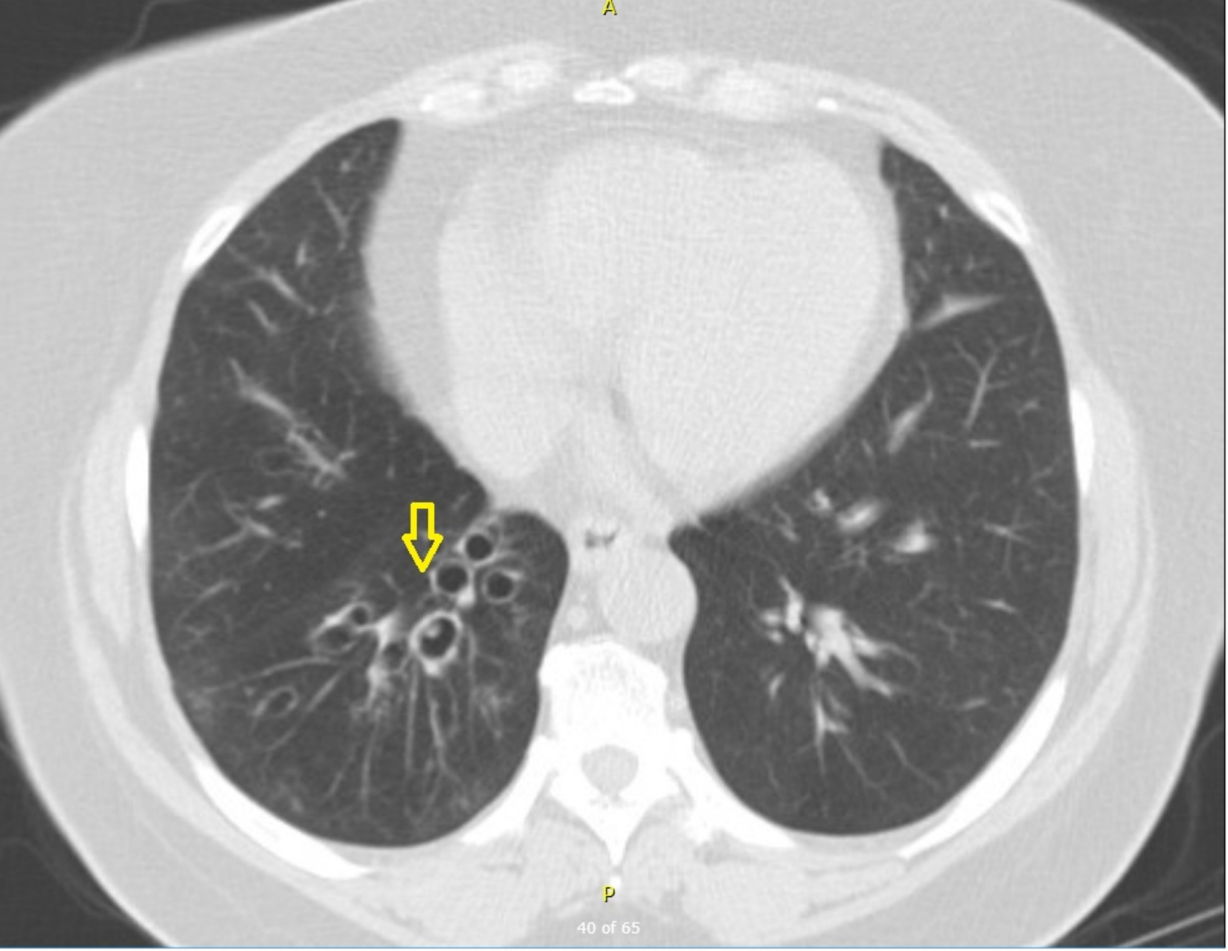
Computed tomography of the chest. Axial view of computed tomography of the chest at the level of lower lobes indicating bronchiectatic changes of the airway (arrow).
